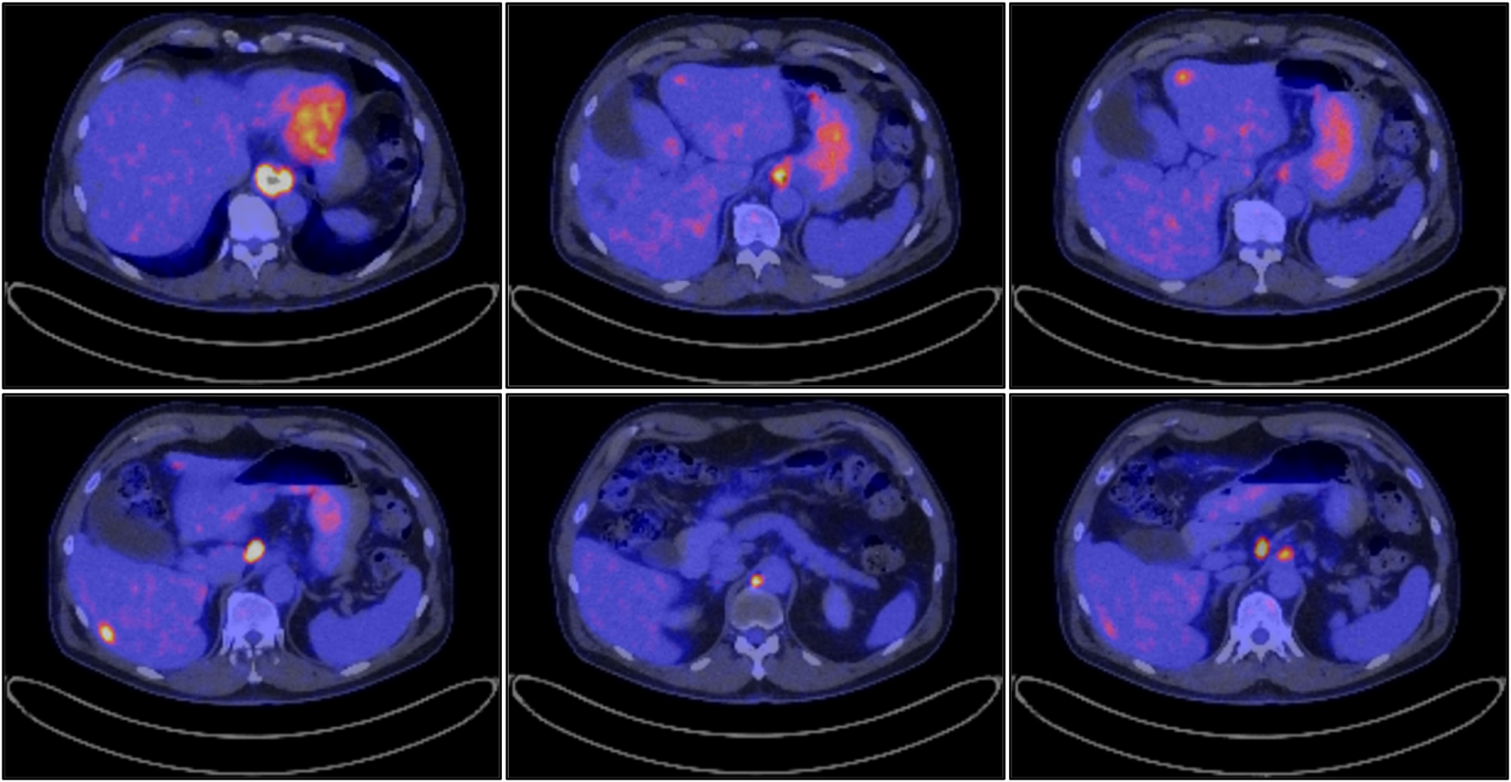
PET-CT showing mediastinal lymph node metastasis, hepatogastric lymph node metastasis and liver metastasis in segment II (8 mm), III (13 mm) and IV (12 mm and 5 mm) at the diagnosis. Images are cited with patient’s consent.
